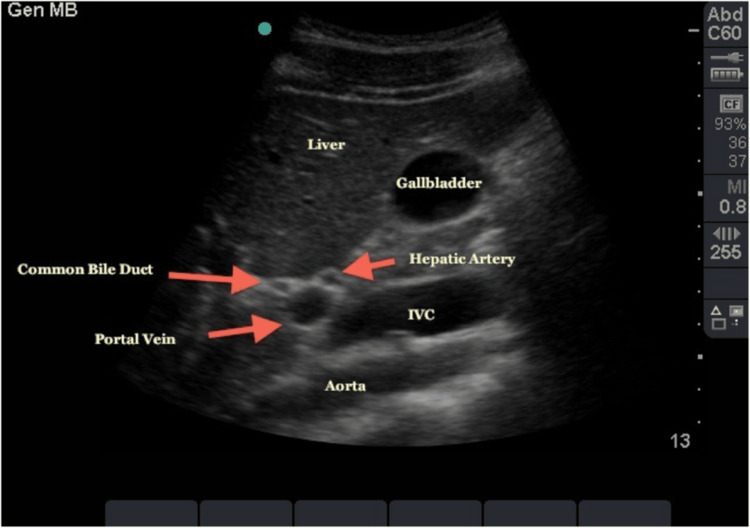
Ultrasound scan of the biliary system showing normal gallbladder and bile ducts. IVC: Inferior vena cava Source: http://www.em.emory.edu/ultrasound/Image
